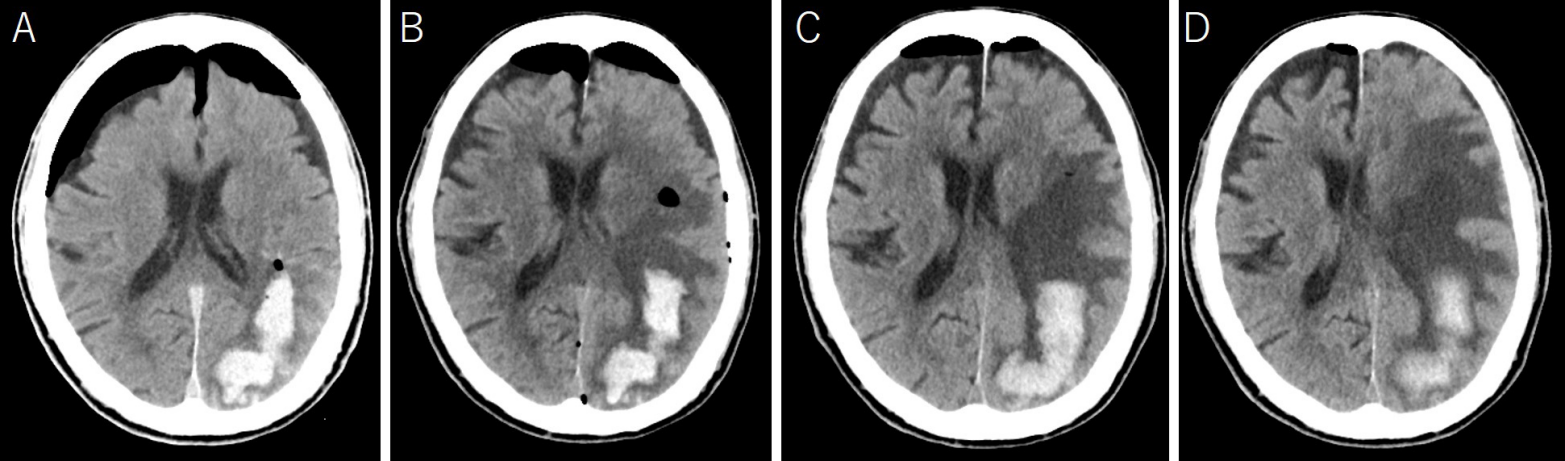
Serial computed tomography (CT) after the first operation revealed the development of a cystic lesion at hematoma and cyst expansion sites. CT immediately after the operation (A); CT on postoperative day 2 (B); CT on postoperative day 8 (C); CT on postoperative day 13 (D).
